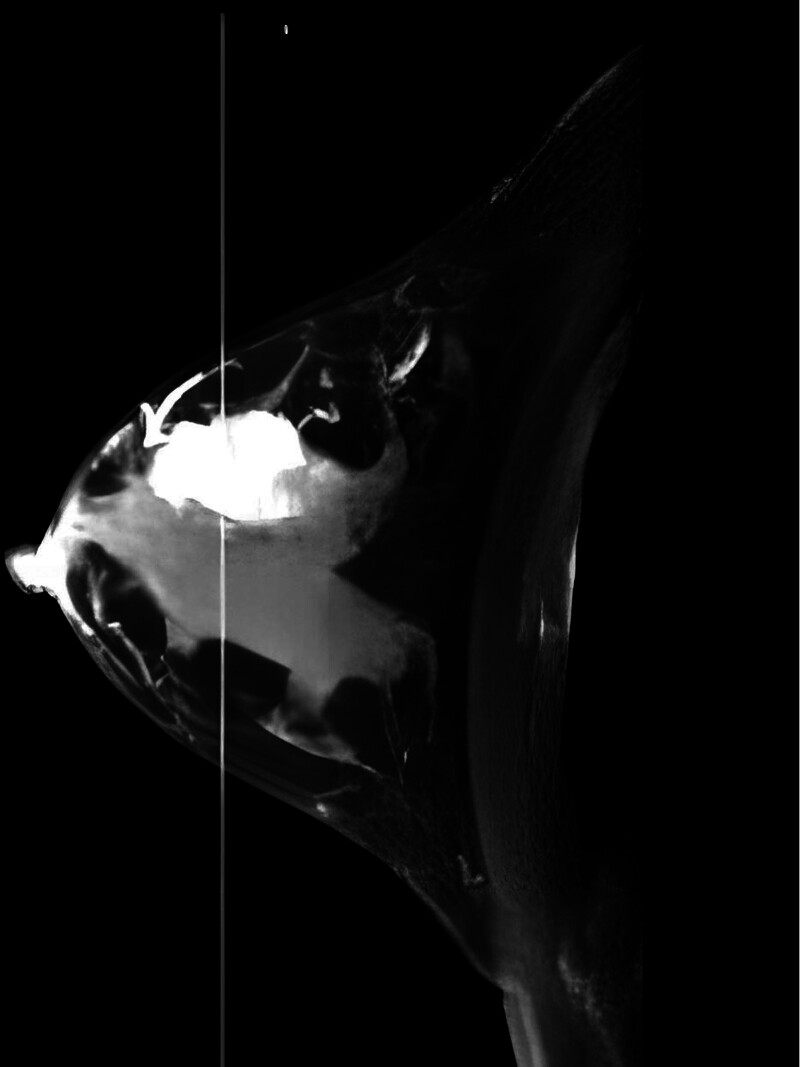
The patient’s breast CT results showed a significantly enhanced mass on the outer side of the right breast. CT = computed tomography.
